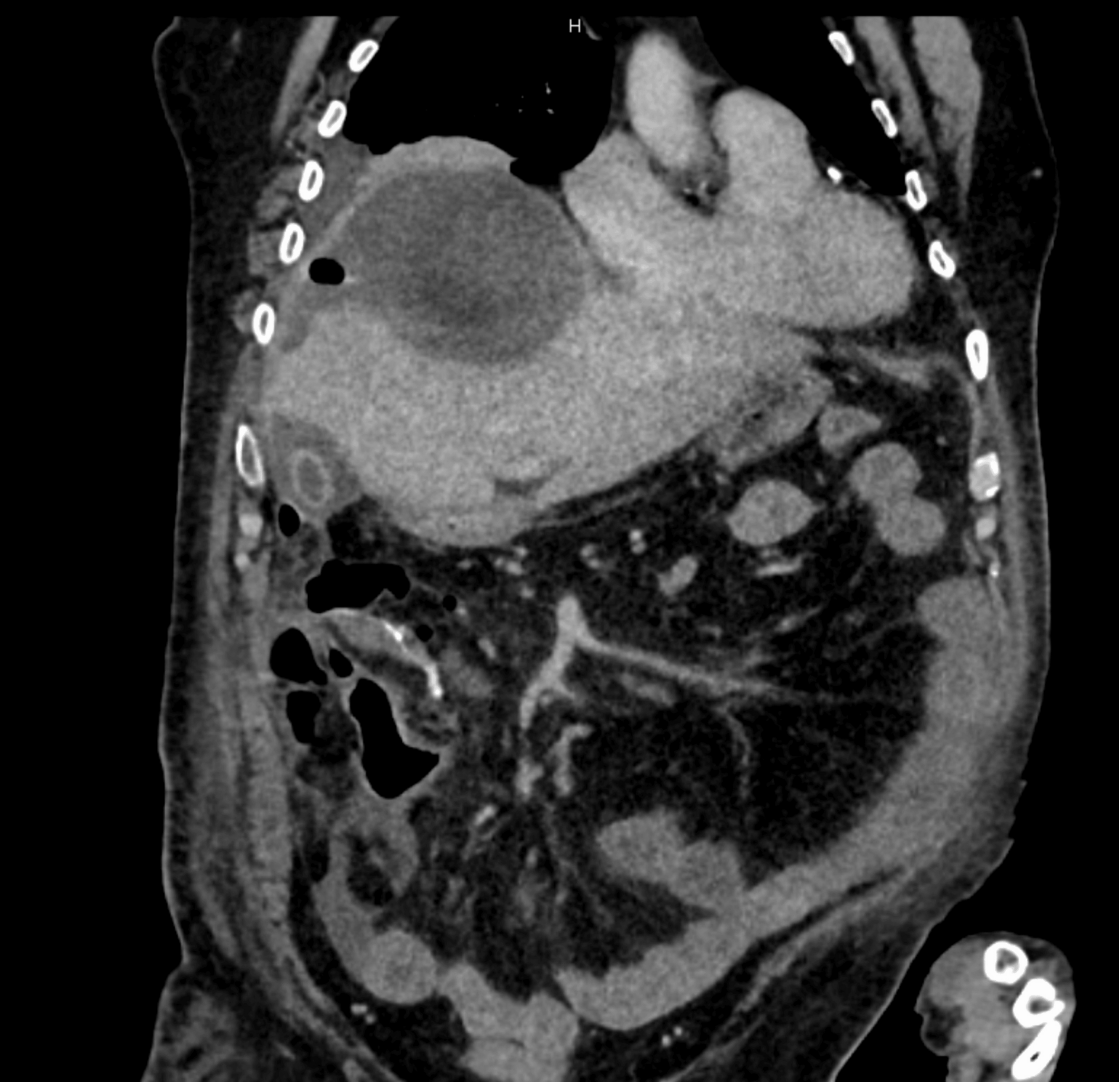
Post-operative Day 9, CT abdomen and pelvis showing a subdiaphragmatic collection. CT: computed tomography.
